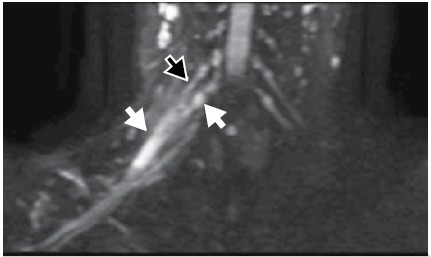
Magnetic resonance imaging, patient 1. Diffusion-weighted neurography - reconstruction in the coronal plane: Thickening and increased signal of the C5 roots (black arrow) and C6 roots (tip of arrow) and upper trunk (white arrow), corresponding to the lesion in continuity.
